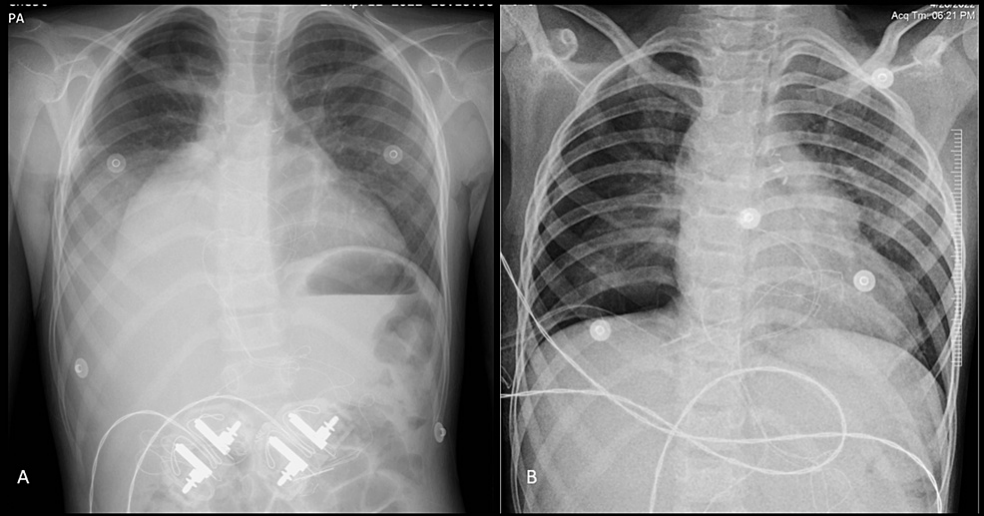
Day 4 chest X-ray showing significant right-sided pleural effusion (A); chest X-ray after right ICD insertion on day 5 (B) ICD: intercostal drainage
